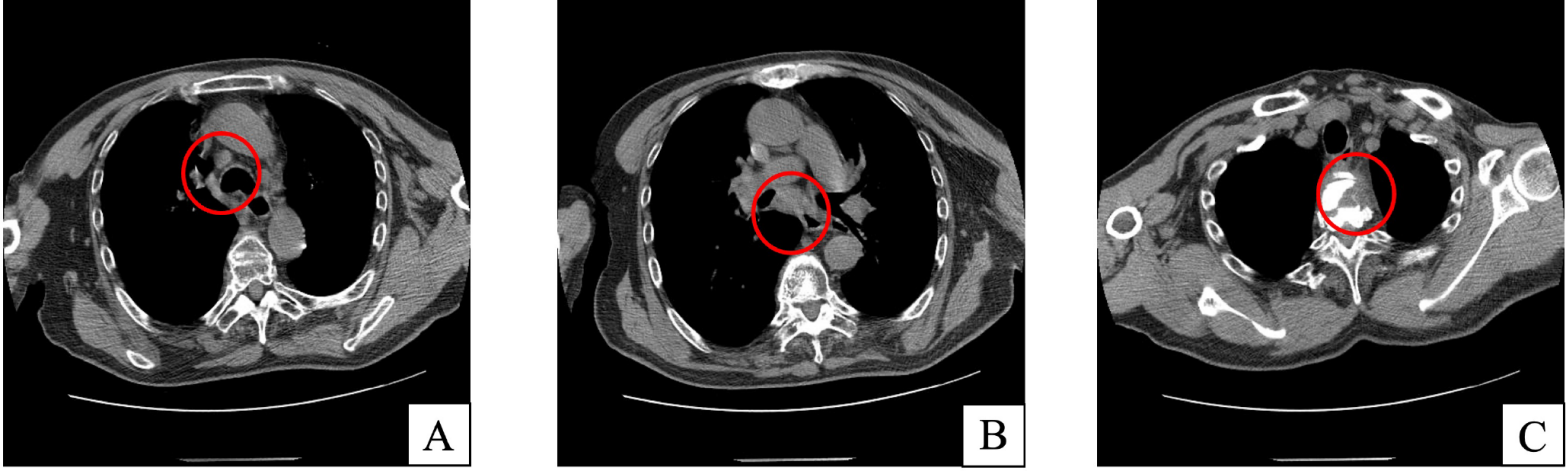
Follow-up chest CT images. Mediastinal window chest CT obtained on December 13, 2024, demonstrated a paravertebral mass-like lesion in the superior mediastinum with adjacent vertebral bone destruction, showing marked improvement compared with the previous examination performed on August 29, 2024 (A–C).
